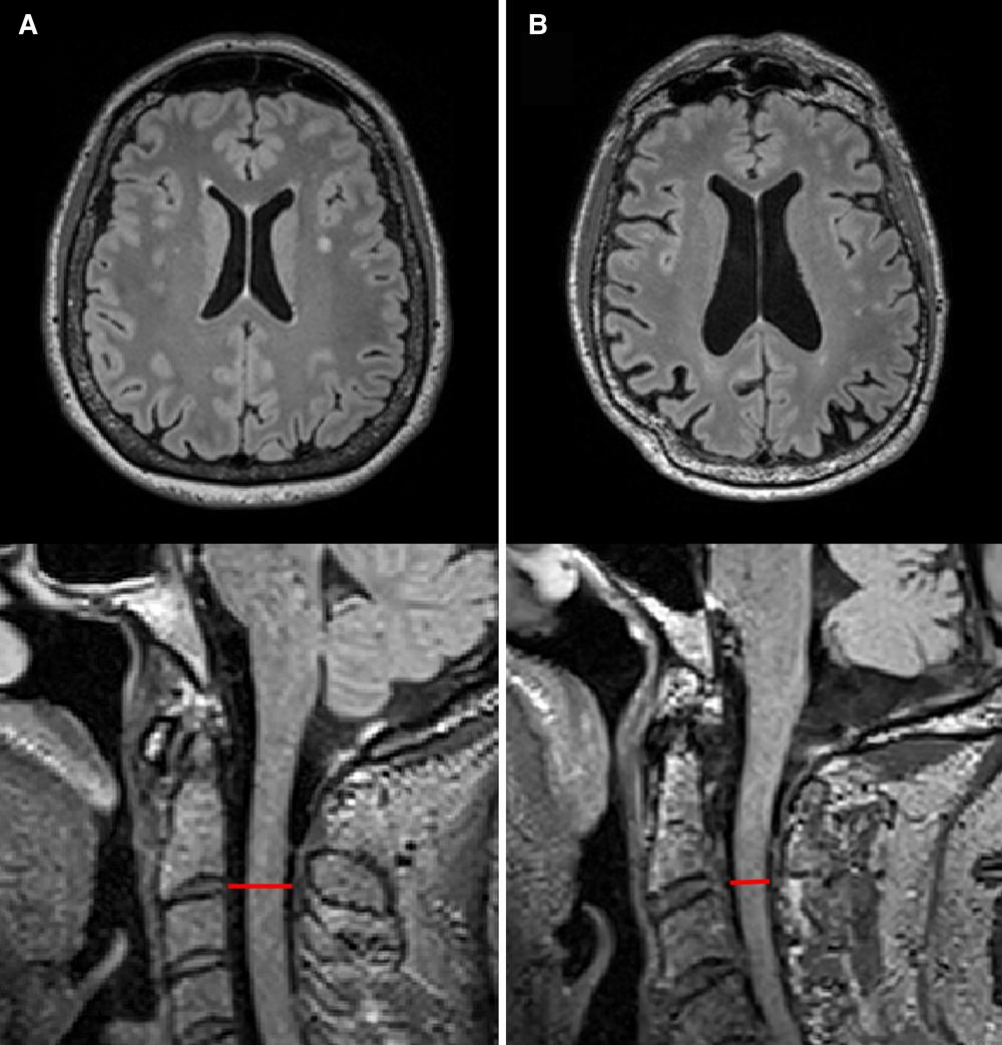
MRI of the brain and cervical spine. Axial T2-weighted FLAIR images of the brain (top row) and midsagittal T2-weighted FLAIR images of the spine at the cervical vertebrae (C) 2/3 level (bottom row). (A) A 51-year-old woman with a larger spinal canal diameter at the C2/3 level of 13.1 mm and non-dilated lateral ventricles. (B) A 69-year-old man with a smaller spinal canal diameter at the C2/3 level of 10.1 mm and larger lateral ventricles.
